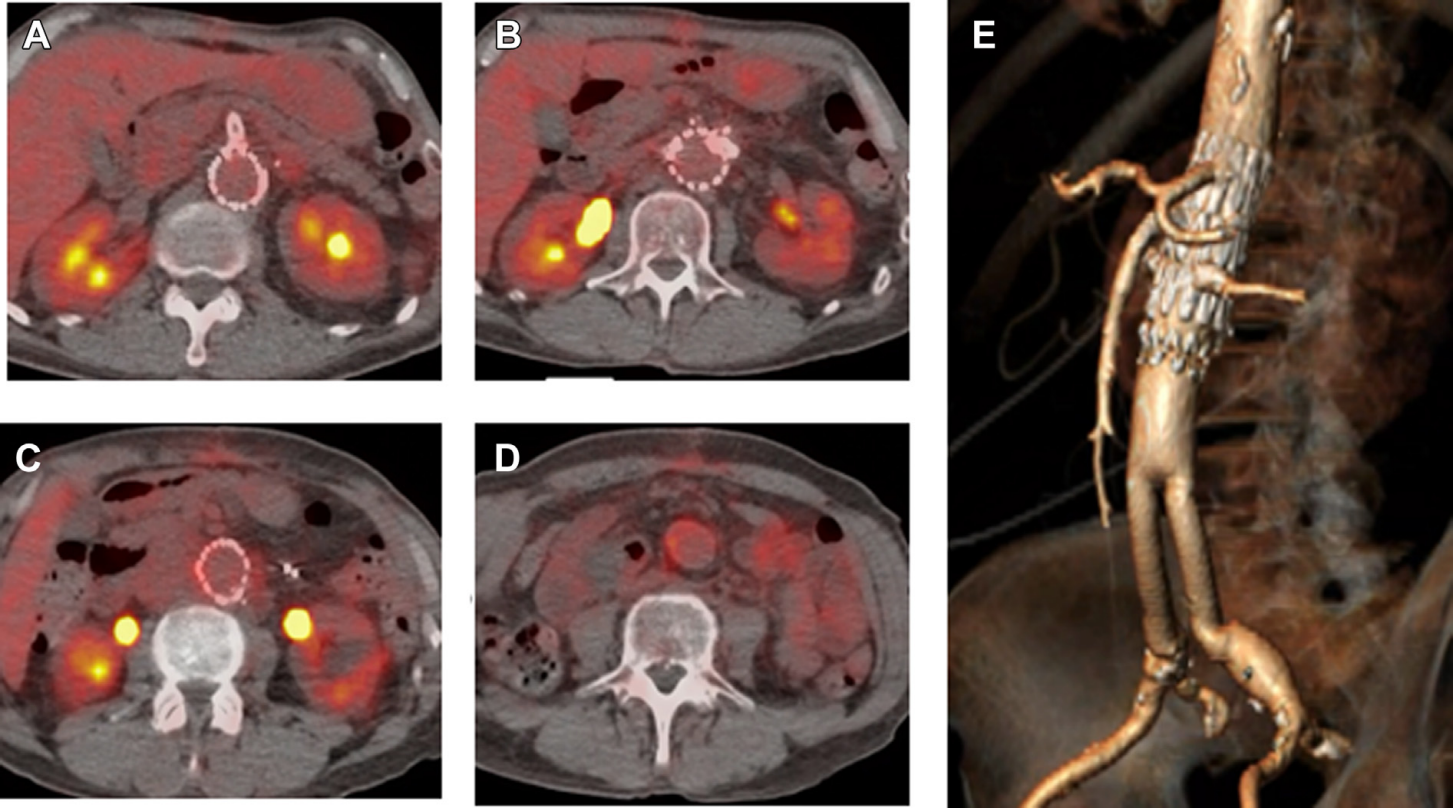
Postoperative positron emission tomography (PET) scans (A-D) showing no uptake and three-dimensional reconstruction computed tomography angiogram showing the residual fenestrated cuff and bifurcated device (E).
